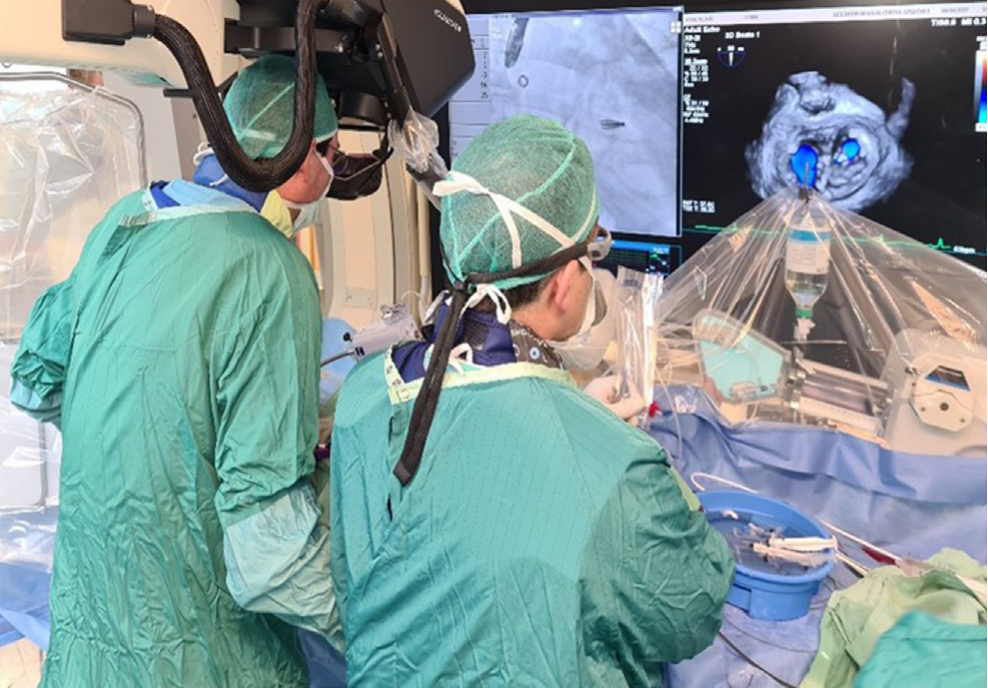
Intraprocedural use of HOLOSCOPE-i (RealView Imaging, Yokneam, Israel) during a mitral valve intervention guided by 3-dimensional transesophageal echocardiography.
